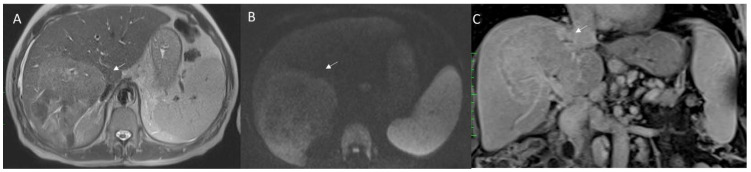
Cholangiocarcinoma patient, classified as LR-M according to LI-RADS, due to targetoid appearance (arrow) in T2-W (A) sequence, in (B) DWI, and (C) late phase of contrast study.
